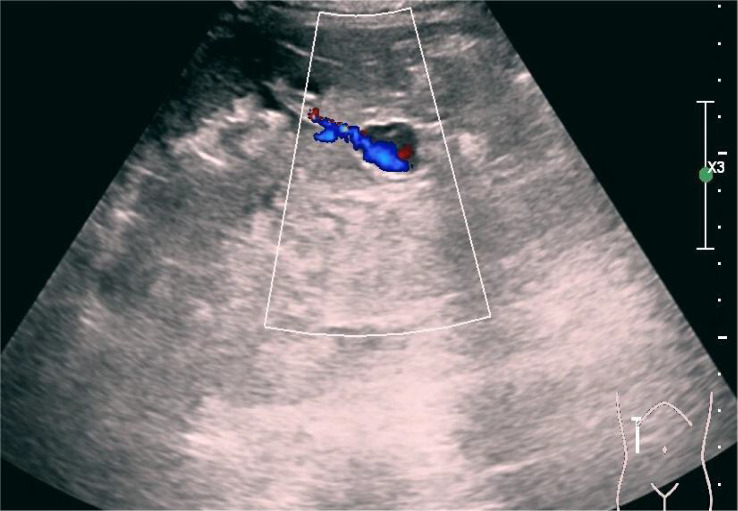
Color Doppler ultrasound after puncture revealing hemorrhagic foci (longitudinal section).
